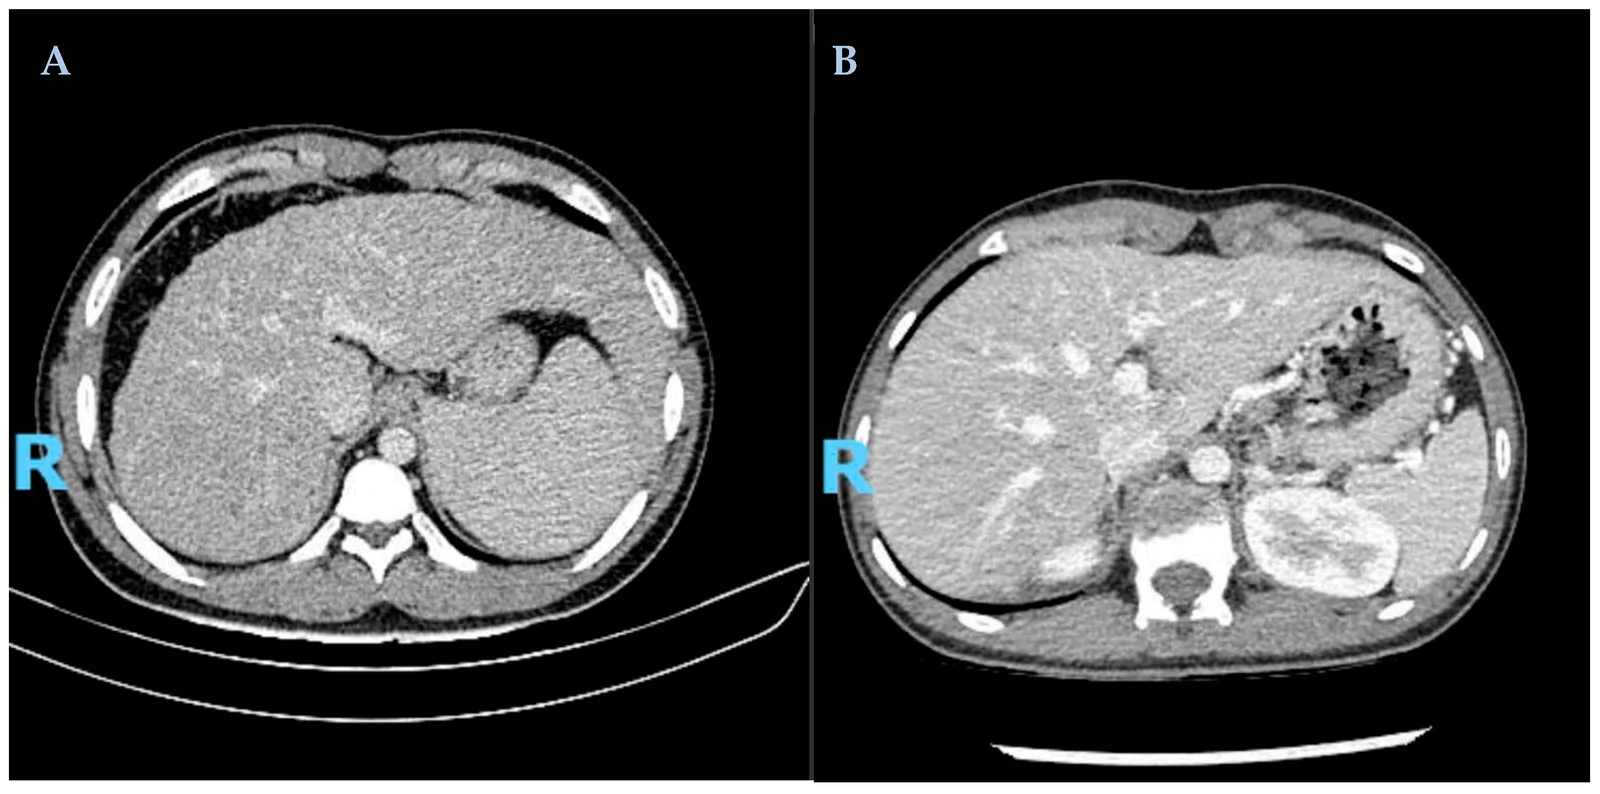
Abdominal CT showing (A) cirrhosis in Case 1 and (B) normal liver in Case 2.
